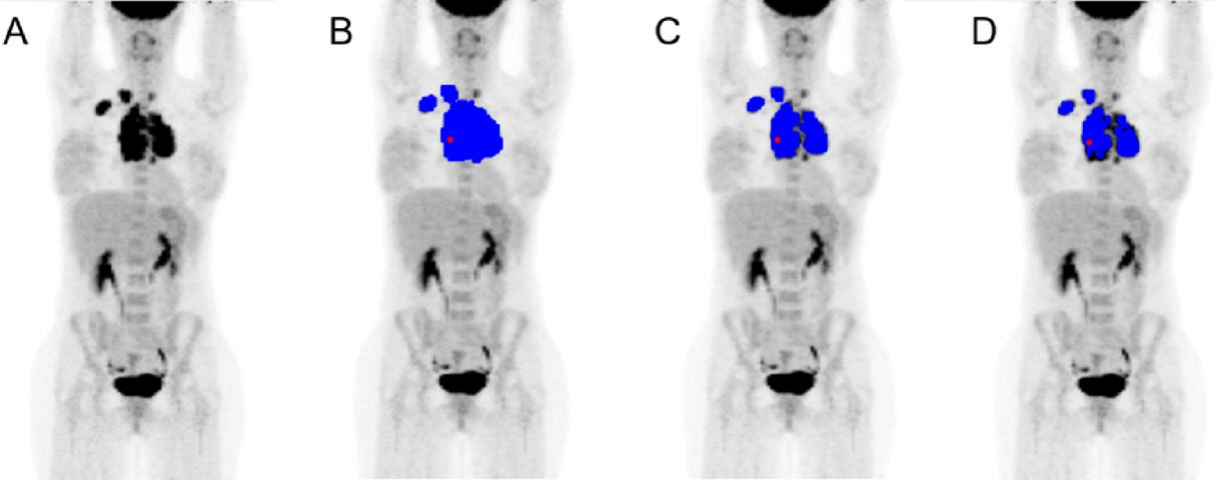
Threshold comparison using BI, (A) baseline, (B) 2.5 SUV, (C) 41% SUV_max_ and (D) 50% SUV_max_.
